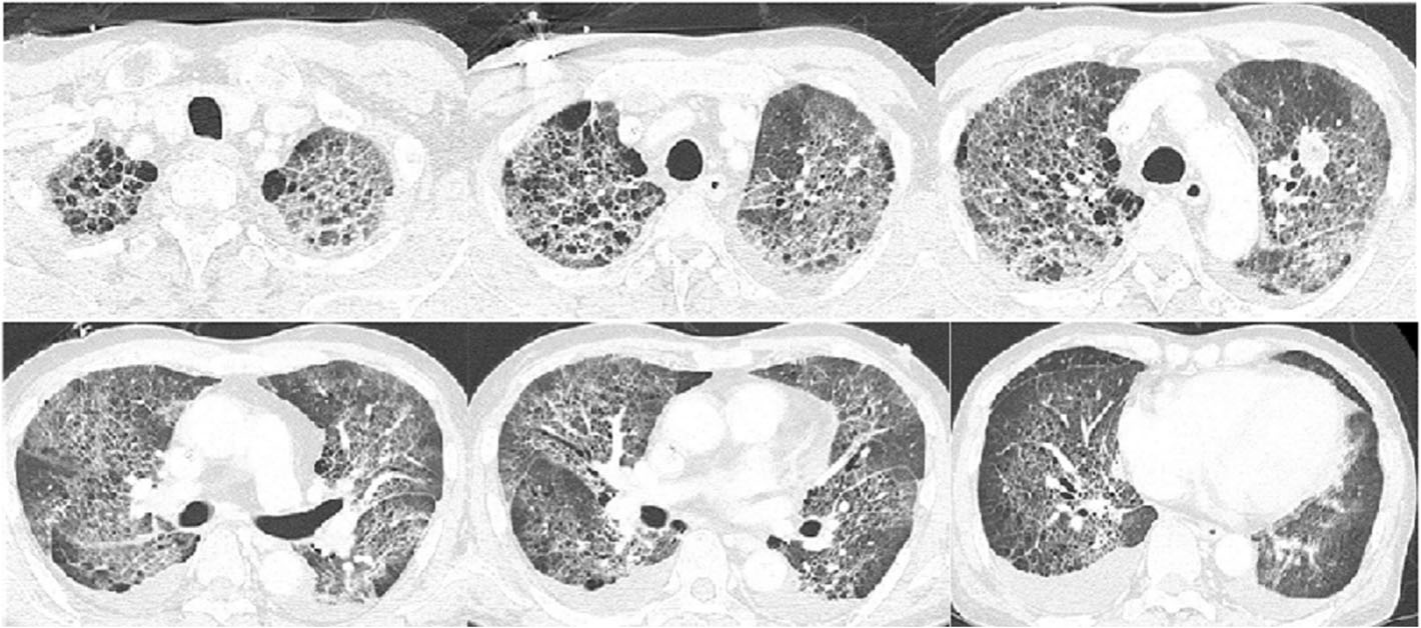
Computed tomography scan showing features of interstitial lung disease
